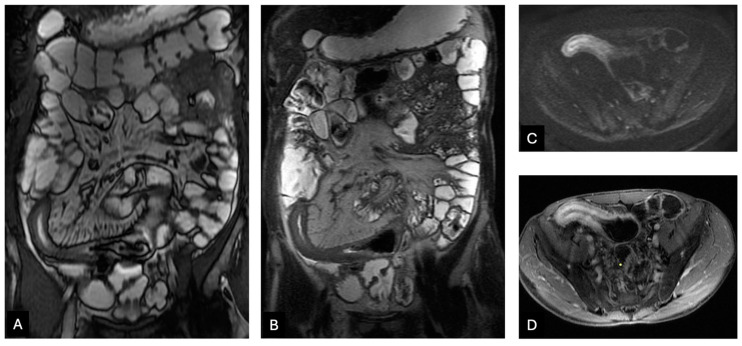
A 49-year-old man with Crohn’s disease involving the neo-terminal ileum. Coronal FIESTA (A) and SSFSE T2WI (B) show a thickened neo-terminal ileum with an increased T2 signal. Axial DWI (*b* = 1000) (C) image at the same level reveals increased intramural signal, indicating restricted diffusion, and the post-contrast axial T1WI (D) demonstrates intensely, layered pattern enhancement (mural stratification), consistent with active inflammation.
